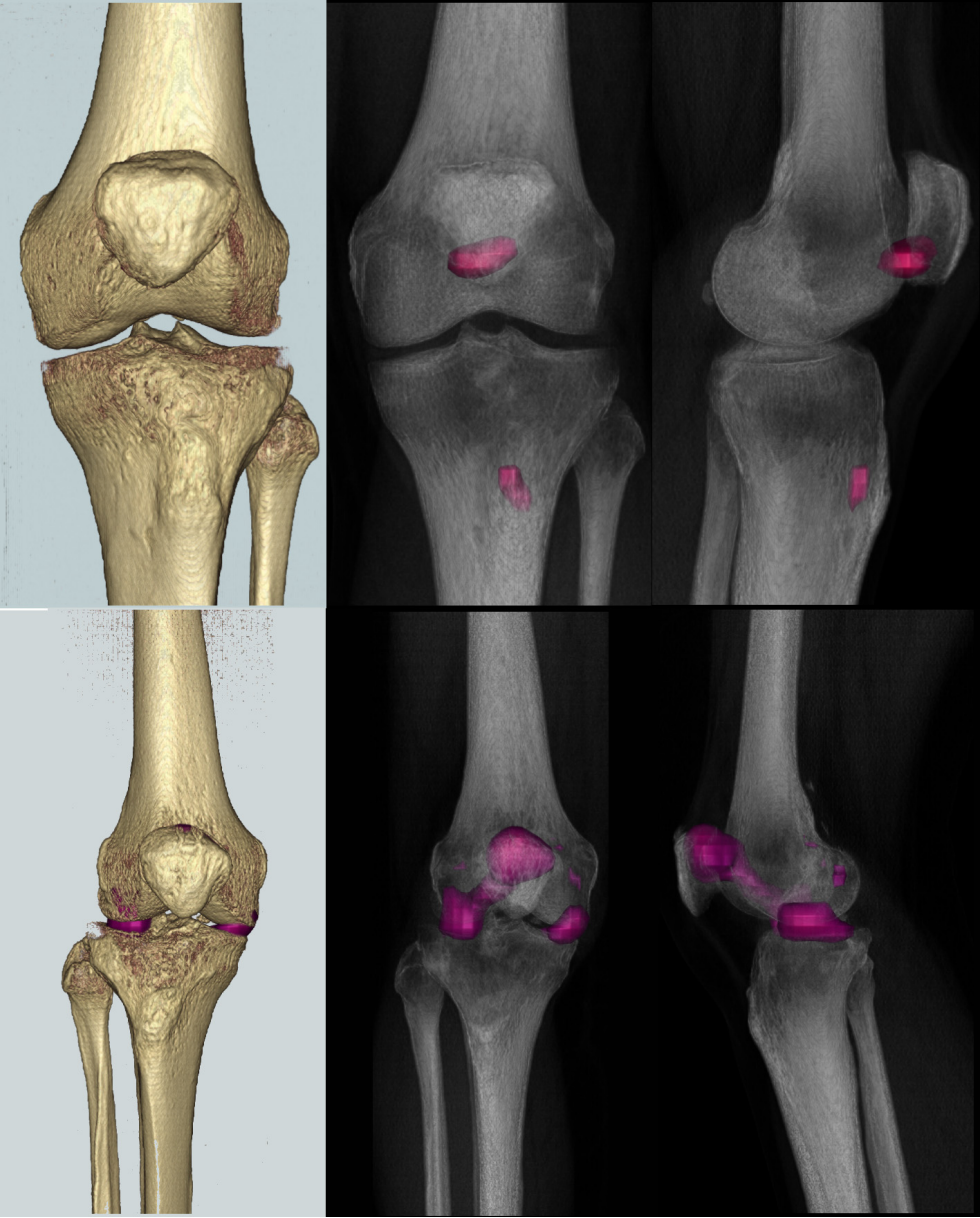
3D reconstructions of SPECT and bone surface. The SPECT hot spots are demonstrated at our chosen SPECT threshold (three times mean of the reference region) as a 3D surface reconstruction (left) and radiolucent (right) views (patient 1 top, patient 2 bottom row).
